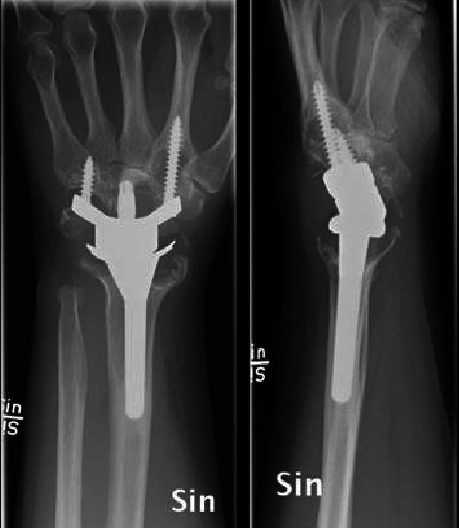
Preoperative radiographs with substantial osteolysis.
